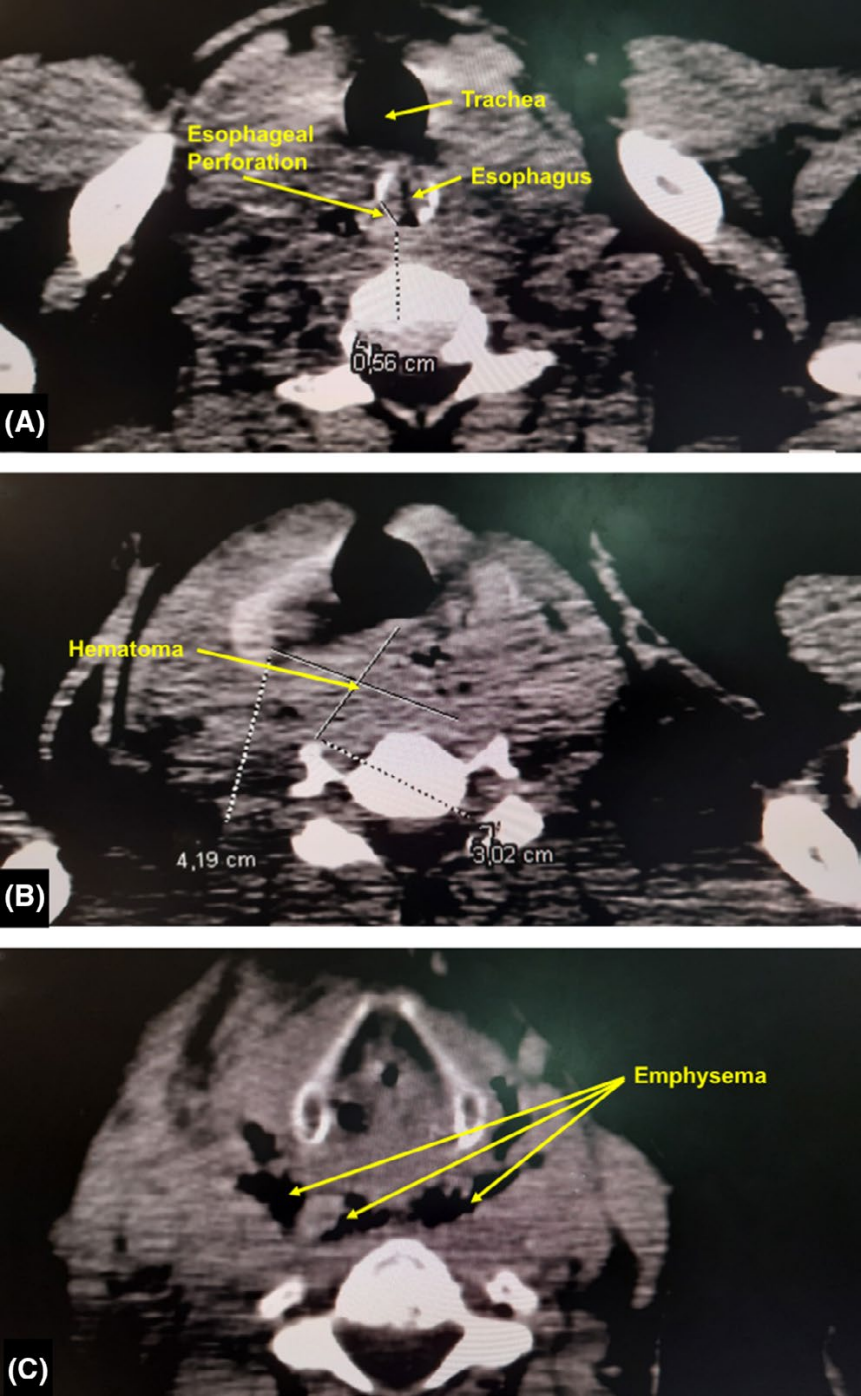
A cervicothoracic computed tomography scan after oral contrast administration showing a perforation (A) in the cervical esophagus, a hematoma (B) of the visceral space of the neck, and an emphysema (C) of the retropharyngeal space
